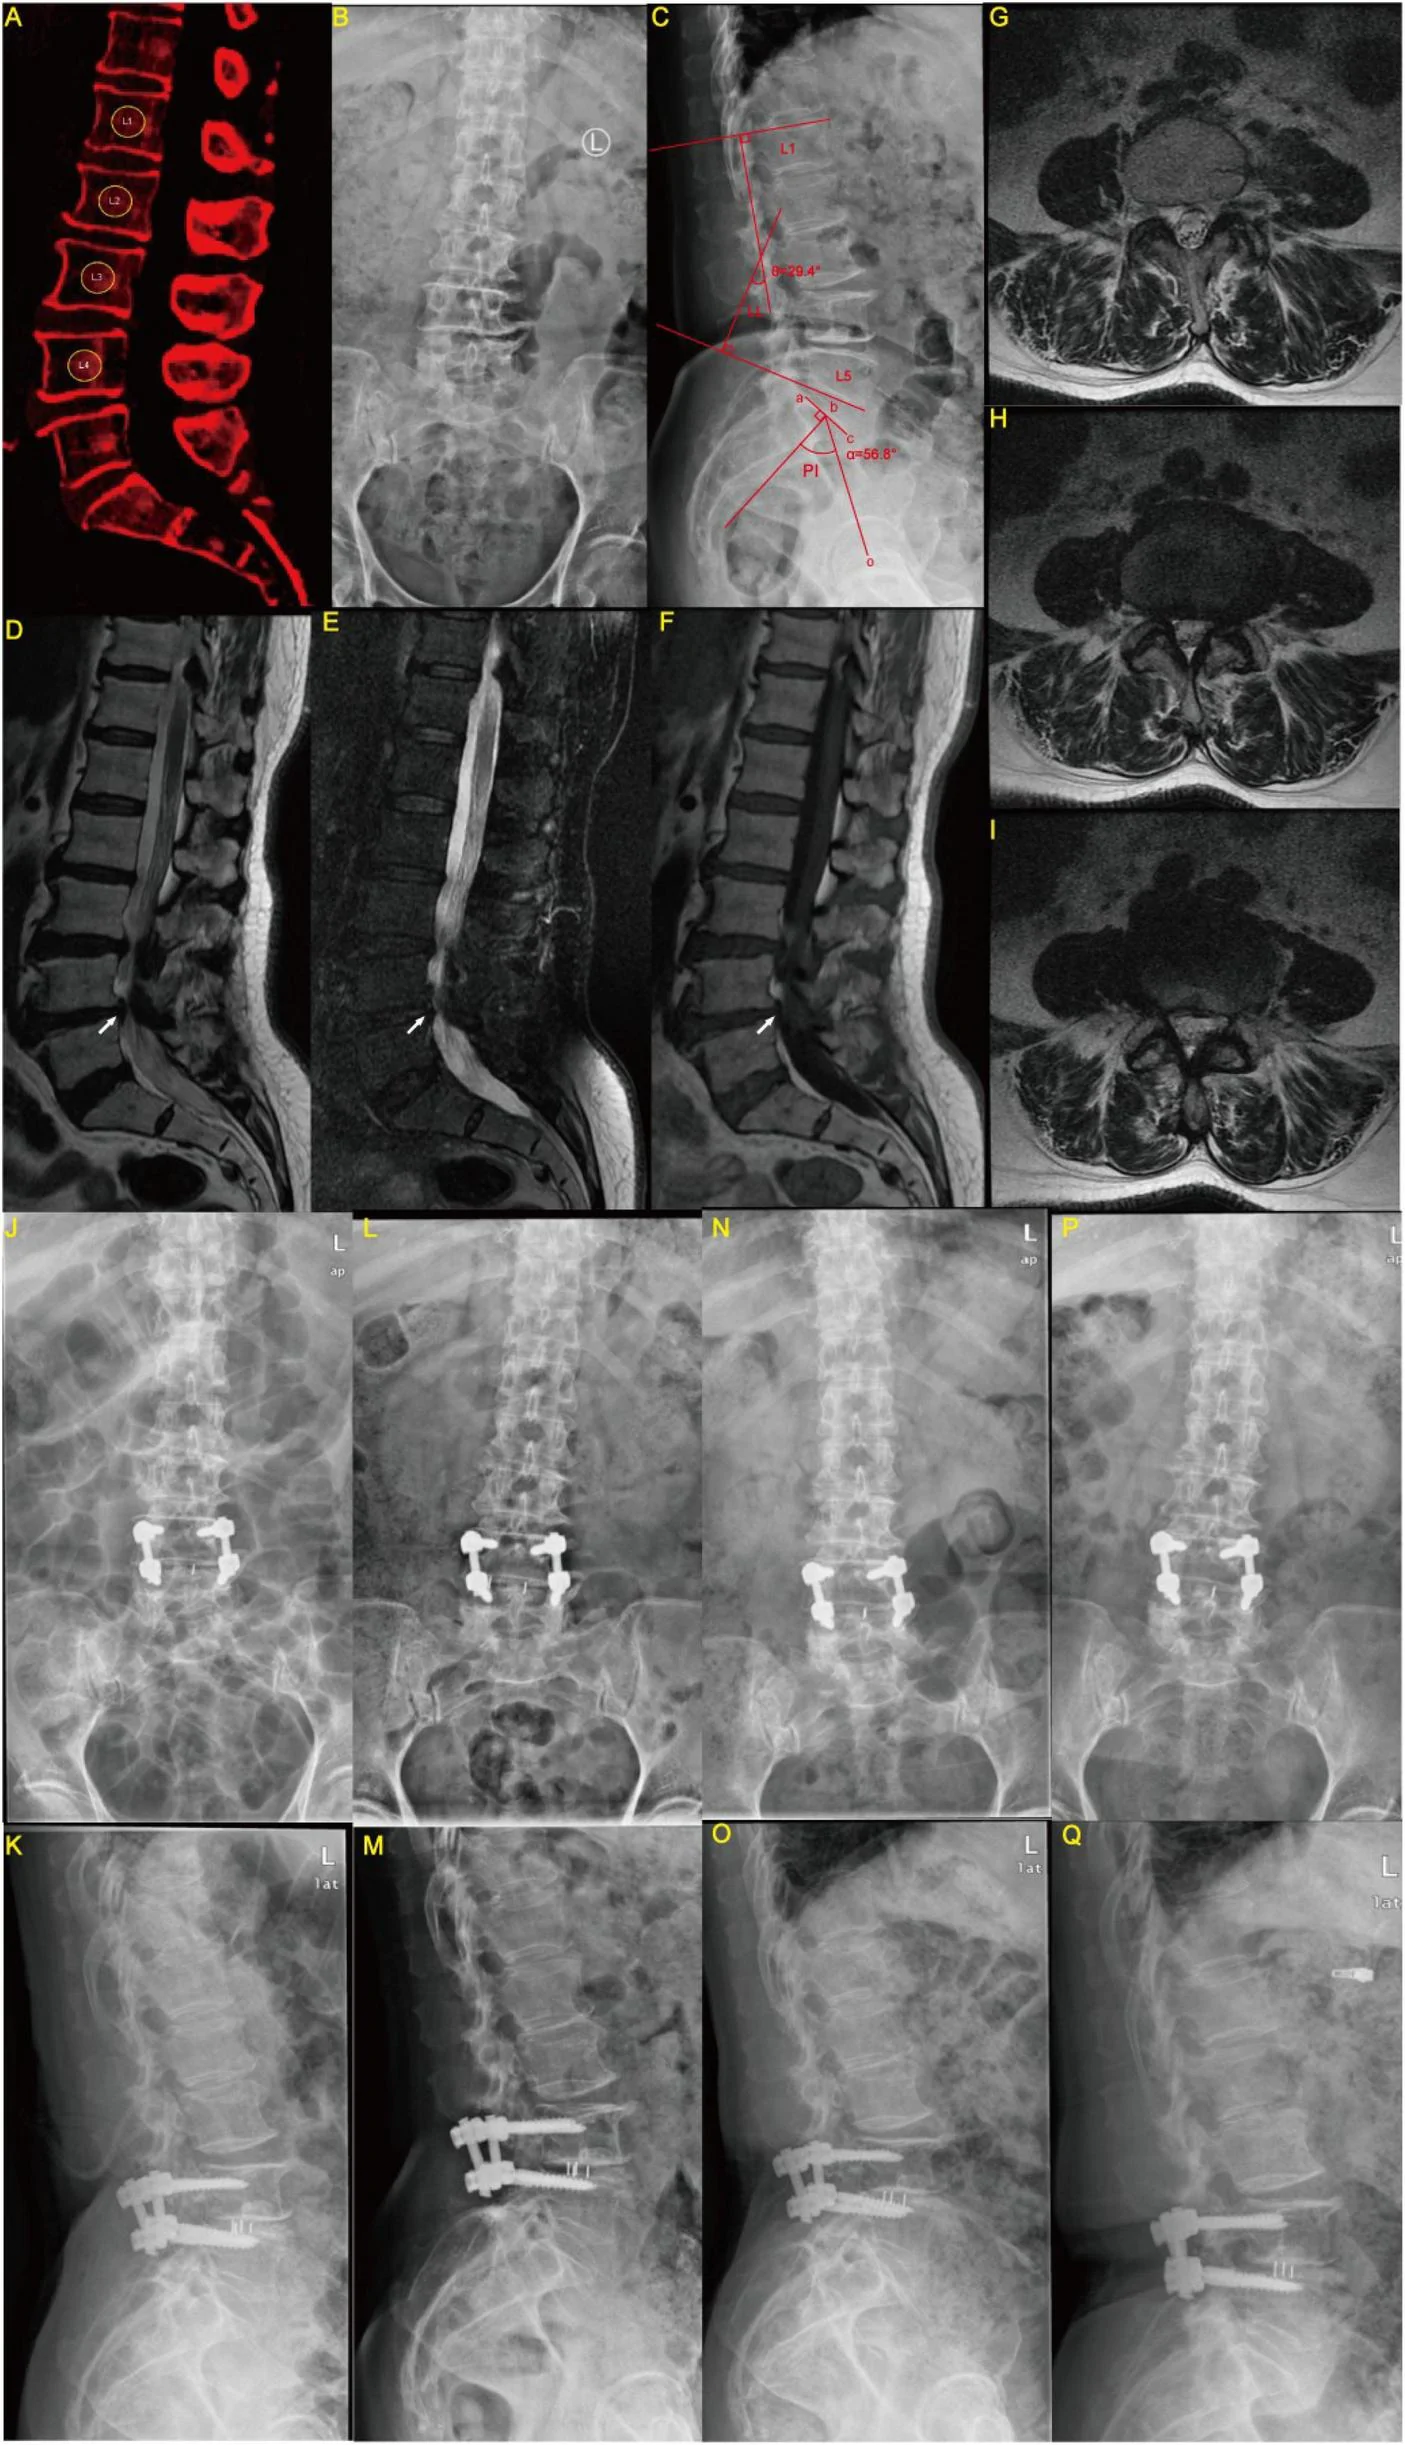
Preoperative imaging evaluation and postoperative follow-up imaging data of a 62-year-old female patient with osteoporosis and screw loosening after lumbar disc herniation surgery. (A) Sagittal reconstructed CT image of the lumbar spine. The region of interest (ROI) was delineated on the L1–L4 vertebral bodies using ImageJ software for bone mineral density measurement, with a T-score of −3.0. (B) Preoperative anteroposterior lumbar radiograph. (C) Preoperative lateral lumbar radiograph. The L1–L5 lumbar lordosis angle was defined as the angle between the perpendicular lines of the superior endplate tangents of the L1 and L5 vertebral bodies, with a measured value of 35.0°. The pelvic incidence (PI) was measured according to the Duval-Beaupère definition. Specifically, the sacral endplate was defined as segment *ac*, and point *b* represented its midpoint. The PI (α angle) was the angle between the perpendicular line of the sacral endplate at point *b* and the line connecting point *b* to the center of the bilateral femoral heads. All angular measurements were performed using the PACS measurement tool with a precision of 0.1°. (D–F) Sagittal MRI images of the lumbar spine. (D,E) T2-weighted images, and (F) a contrast-enhanced T1-weighted image. The white arrows indicate L4/5 segment lumbar disc herniation. (G–I) Axial T2-weighted MRI images at different layers of the L4/5 segment. (J,K) Immediate postoperative anteroposterior and lateral lumbar radiographs, showing the initial positioning of pedicle screws and interbody fusion cage at the L4/5 segment. (L,M) Lumbar anteroposterior and lateral radiographs at 3 months postoperatively, demonstrating stable internal fixation. (N,O) Lumbar anteroposterior and lateral radiographs at 6 months postoperatively, showing signs of screw loosening. (P,Q) Lumbar anteroposterior and lateral radiographs at 12 months postoperatively, showing progressive screw loosening and partial loss of lumbar spinal alignment.
